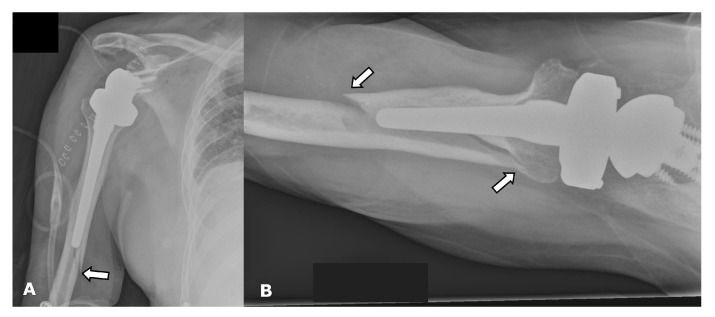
(A). Frontal radiograph in a patient immediately post-operative from RTSA shows a periprosthetic humeral fracture (arrow). (B). Axillary radiograph in a different patient with RTSA shows a periprosthetic fracture along the humeral stem (arrows).
